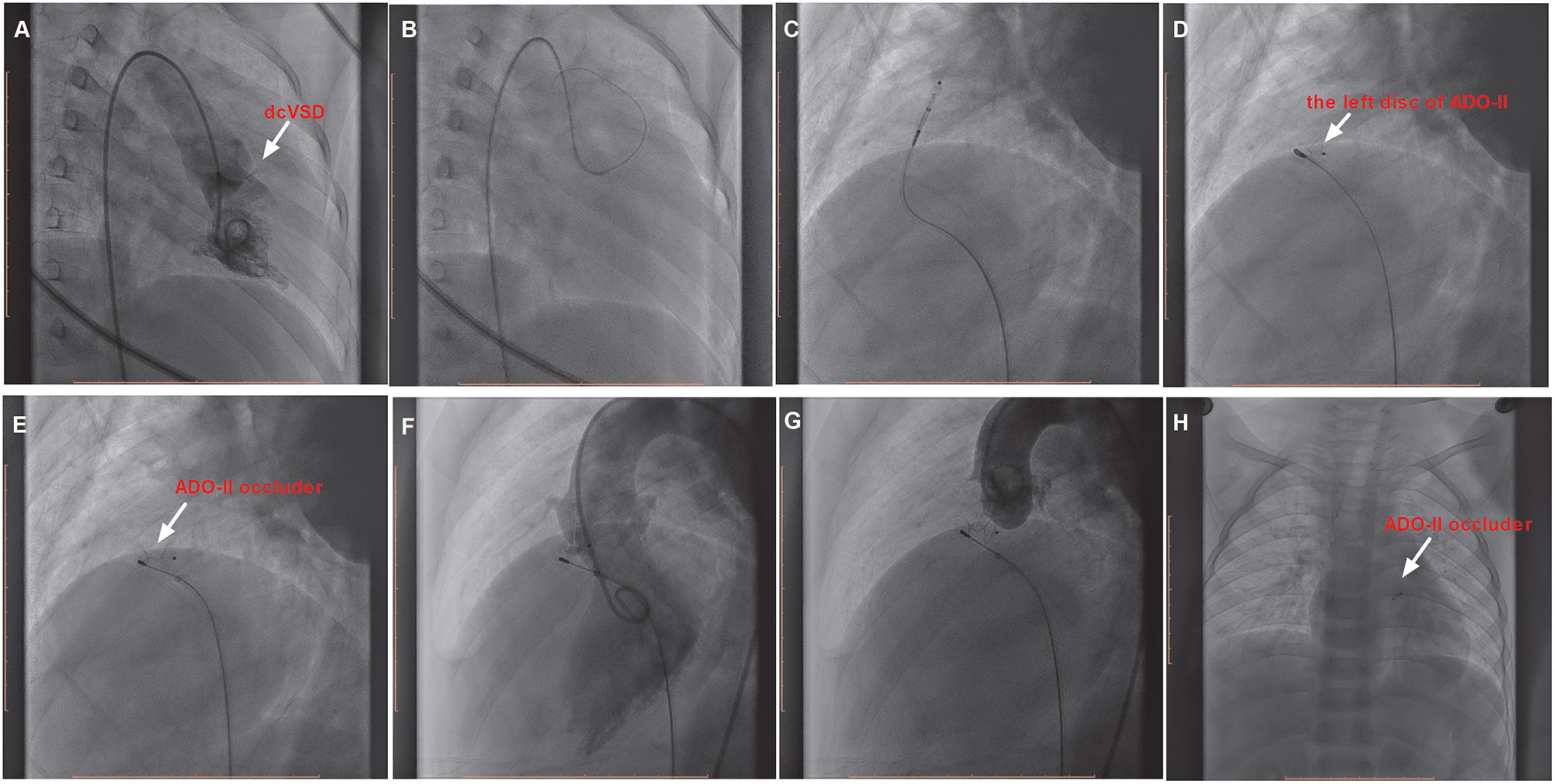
The procedure of transcatheter closure of the dcVSD using the ADO-II device *via* the antegrade approach. (A) Confirms the location of the dcVSD (arrow) with left-to-right shunting on left ventriculography before the procedure. (B–E) Show the whole processes of the transcatheter technique. (F) shows the operational success and no significant RS by left ventriculography, and (G) Shows no AR after device implantation by aortography before releasing the occluder. (H) Demonstrates the good position and configuration of the released occluder under X-ray fluoroscopy. dcVSD, doubly committed subarterial ventricular septal defect; AR, aortic regurgitation; RS, residual shunt.
